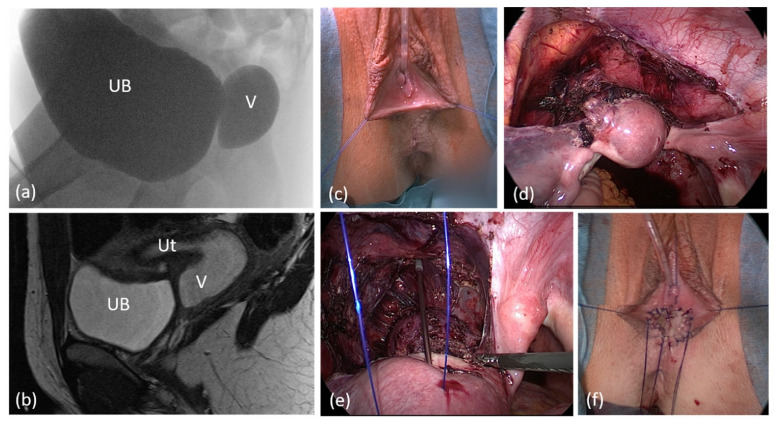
Laparoscopic pull-through in a case of unrecognized cloacal malformation with high urogenital sinus. (a) Genitogram (b) sagittal pelvic MRI. (c) Appearance of Introitus preoperatively. (d), (e) Laparoscopic view after mobilization of the uterus and proximal vagina. (f) Introitus after the vaginal pull-through; the vagina was calibrated with hegar 18, length 9 cm. UB = urinary bladder; V = vagina; Ut = uterus.
